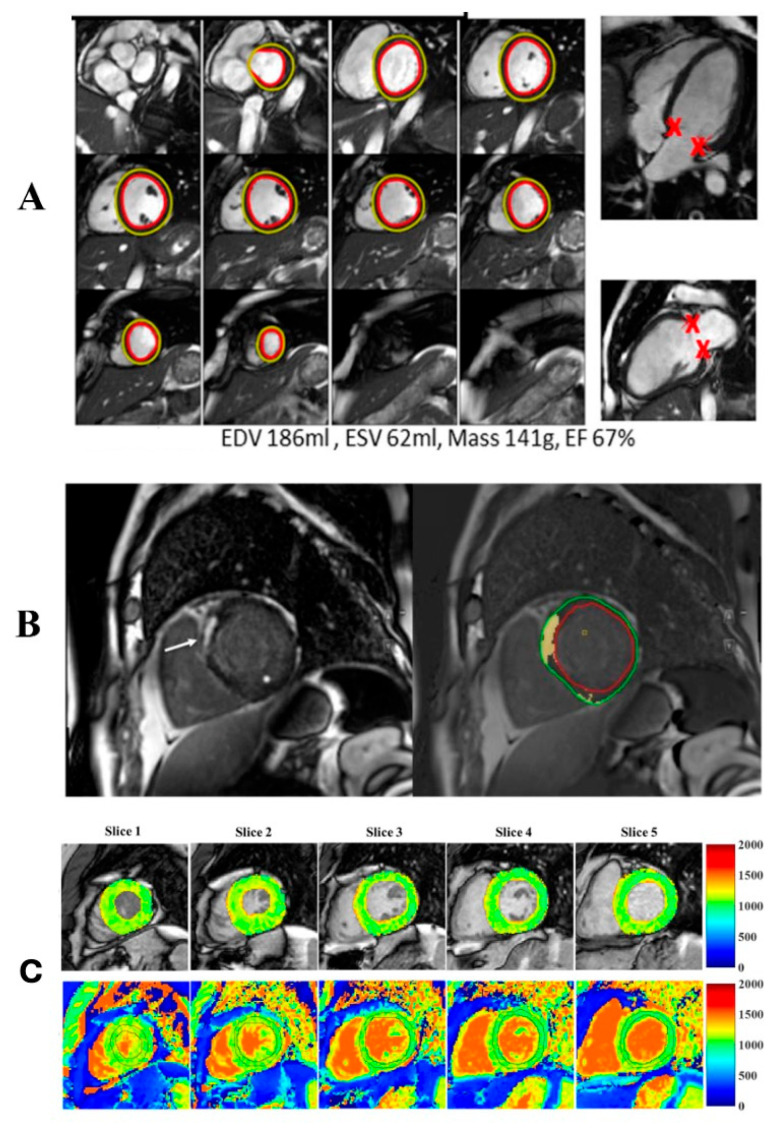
Artificial intelligence applications in cardiac magnetic resonance imaging. AI-based methods are used for automated segmentation, functional quantification, and tissue characterization from routinely acquired CMR datasets. In this figure, AI does not aim to improve raw image quality but to enable efficient and reproducible analysis. (A) Automated delineation of the endocardium (red) and epicardium (yellow) with automated calculation of left ventricular mass, volumes, and ejection fraction. ‘X’ denotes the annotated mitral annular reference point. This approach reduces processing time from approximately 10 min to less than 1 min. Adapted from Davies RH et al. [30]. (B) Quantitative assessment of myocardial fibrosis (late gadolinium enhancement—LGE) in a patient with myocardial infarction. Left: visual depiction of LGE (arrow). Right: automated quantification of fibrosis extent (yellow) in relation to total myocardial mass (between green and red contours). Adapted from Lanzafame LRM et al. [31]. (C) Automated (top row) versus manual (bottom row) quantification of T1 mapping, showing excellent agreement (1091 ± 59 ms vs. 1089 ± 59 ms, respectively). Adapted from Fahmy AS et al., [32].
